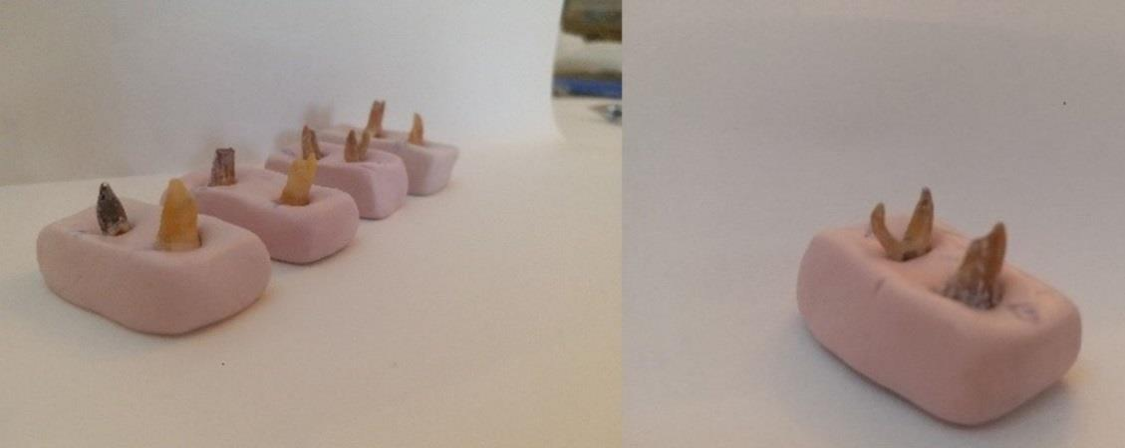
Mounting of the roots in putty to undergo cone beam computed tomography (CBCT)
